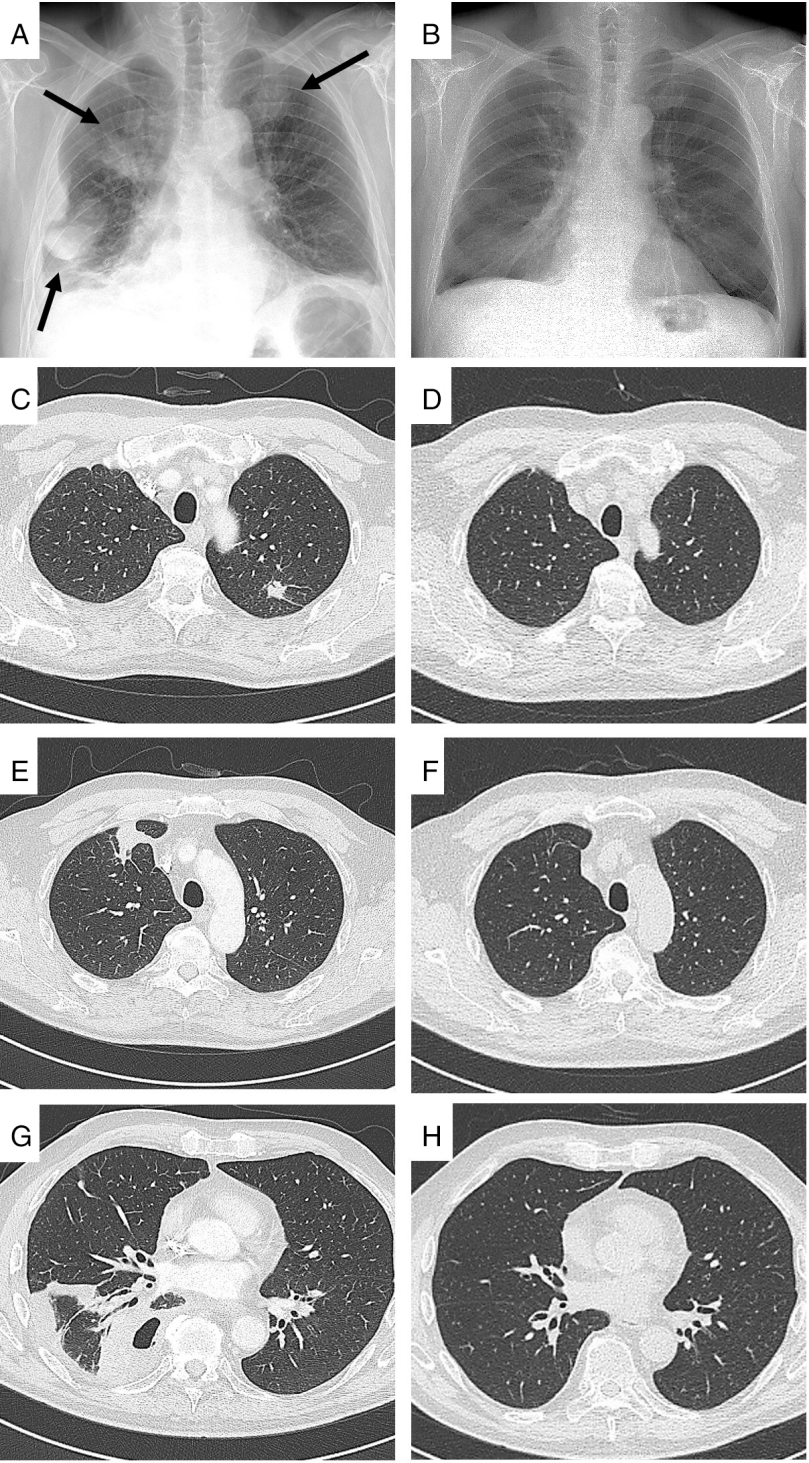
Chest X‐ray (A) and computed tomography (CT) scan (C, E, G) at admission. Chest X‐ray at discharge (B). CT scan at three months' follow‐up (D, F, H).
